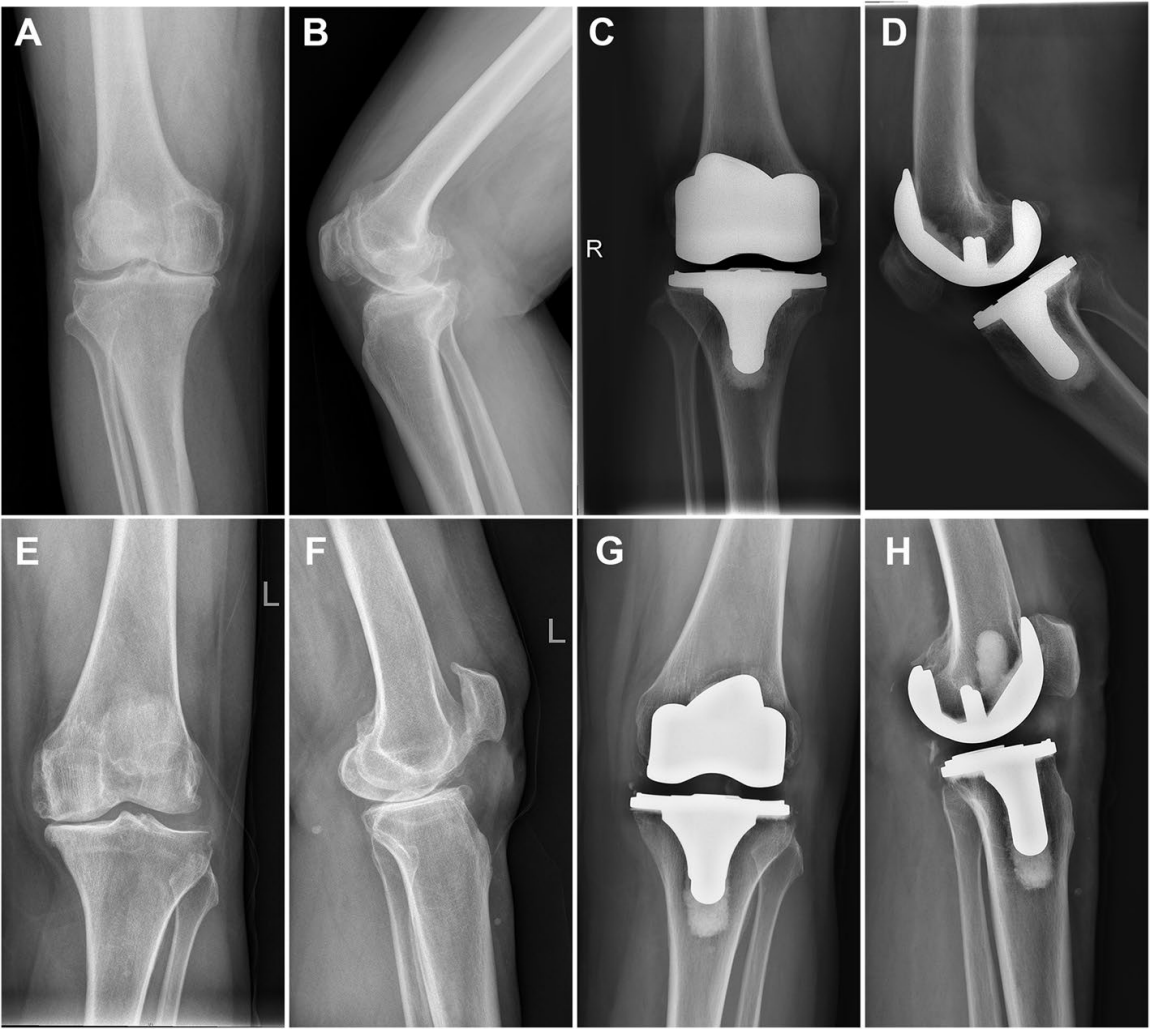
Preoperative and final X-ray of medial-pivotal (MP) insert (panel A-D) and double-high (DH) insert (panel E-H) used in cruciate-retaining TKA
